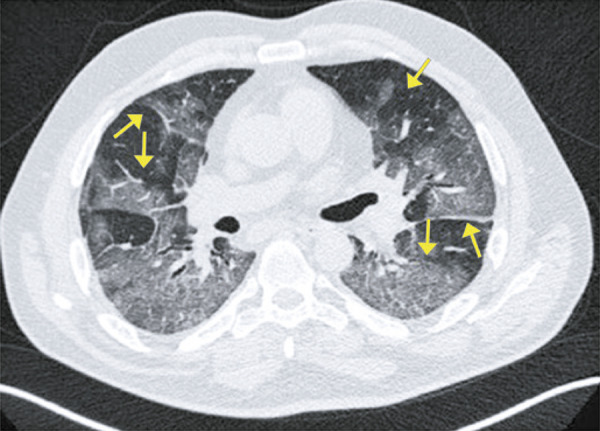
A 63-year-old patient with COVID-19, presenting dyspnea, fever, chills and myalgia for four days. Computed tomography showing ground-glass opacities associated with reticulations (crazy paving pattern)
